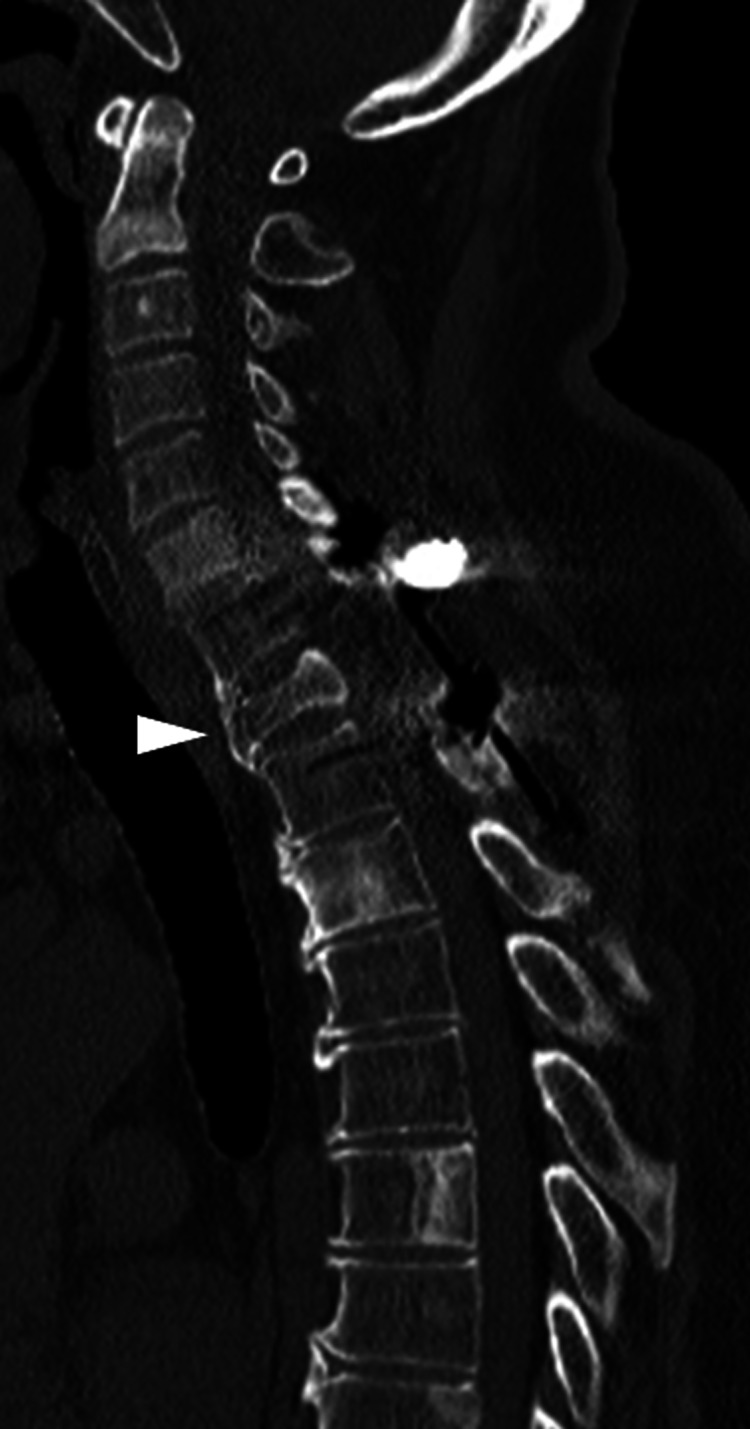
CT images at two years and six months after surgery. Bone union was seen between the T1 and C7 vertebral body with bony bridging (arrowhead).
